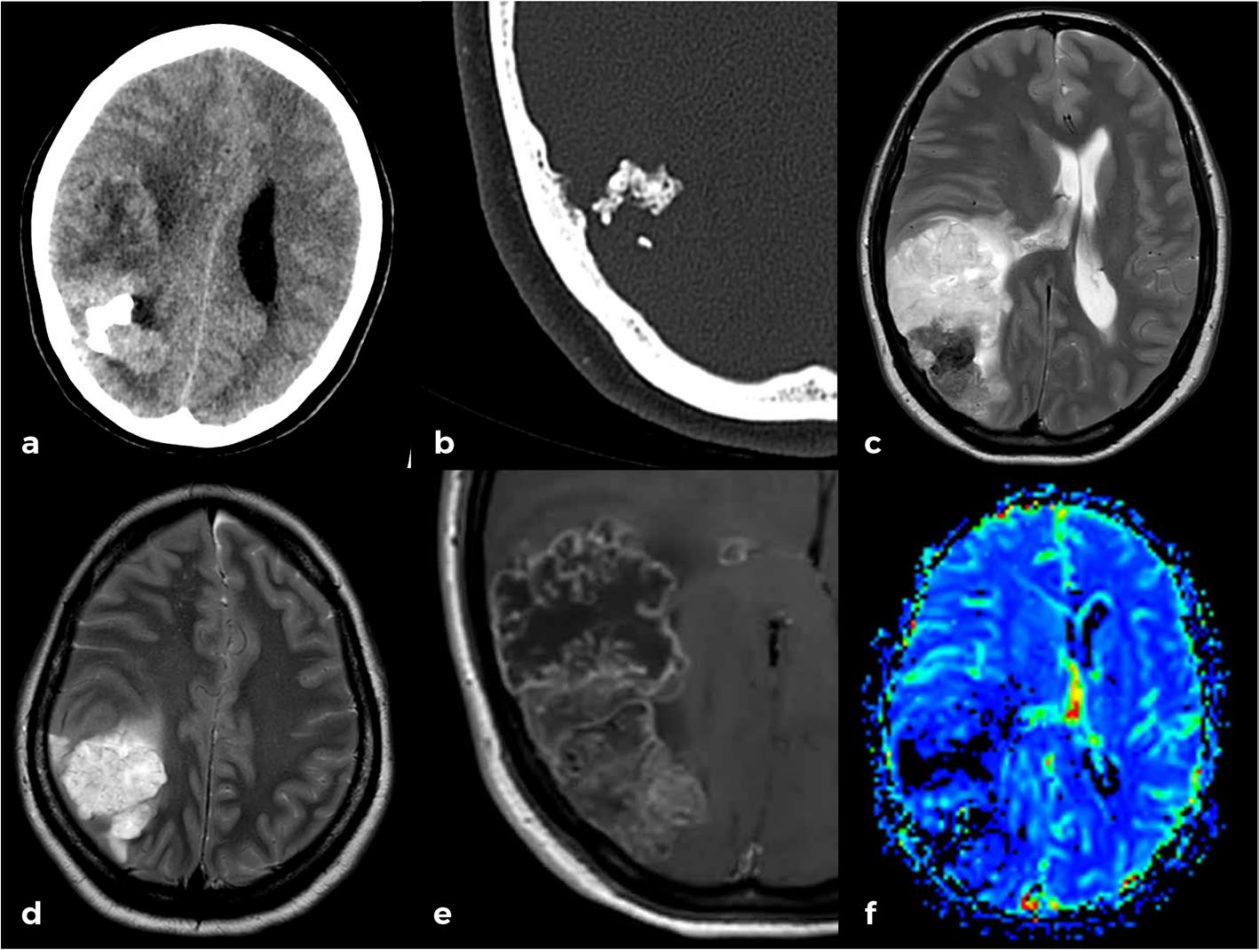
Axial brain-parenchyma (a) and bone windows (b) of brain in non-enhanced CT demonstrating a parietal mass with subtle hyperostosis and internal calcifications. Axial T2 WI (c,d) shows a lobulated mass which is predominantly hyperintense but with some foci of low signal. Significant mass effect and subtle edema is noted. T1-WI postcontrast (e) and axial T2* MRI perfusion (f) images show a heterogeneous enhancement with honeycomb pattern and low CBV of the tumor
